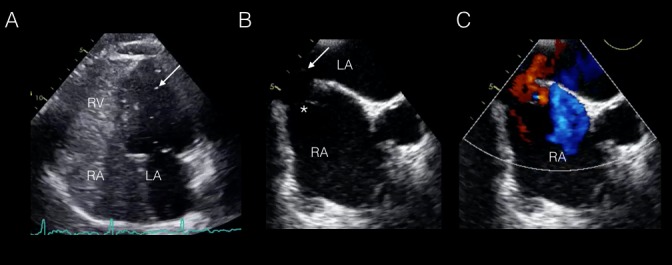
Multimodality Cardiac Imaging with Transthoracic and Transesophageal Echocardiography (A) An apical four chamber view on transthoracic echocardiography demonstrating a grade 1 intracardiac shunt following the administration of agitated saline contrast (arrow); (B-C) A bicaval view on transesophageal echocardiography without and with color Doppler demonstrating bidirectional flow across the inferior sinus venosus defect (arrow) with a prominent Eustachian valve (*). LA:, left atrium; RA: right atrium; RV: right ventricle
